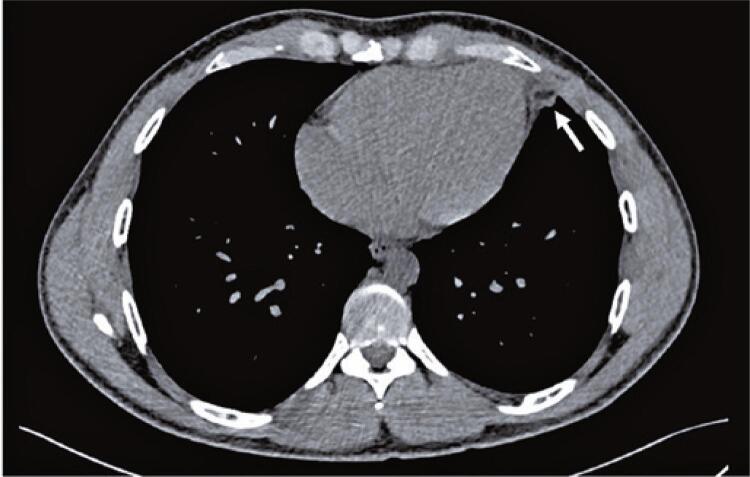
Computed tomography of the chest on axial view evidencing a rounded lesion (arrow) with fat attenuation and variable degrees of densification of the adipose planes
